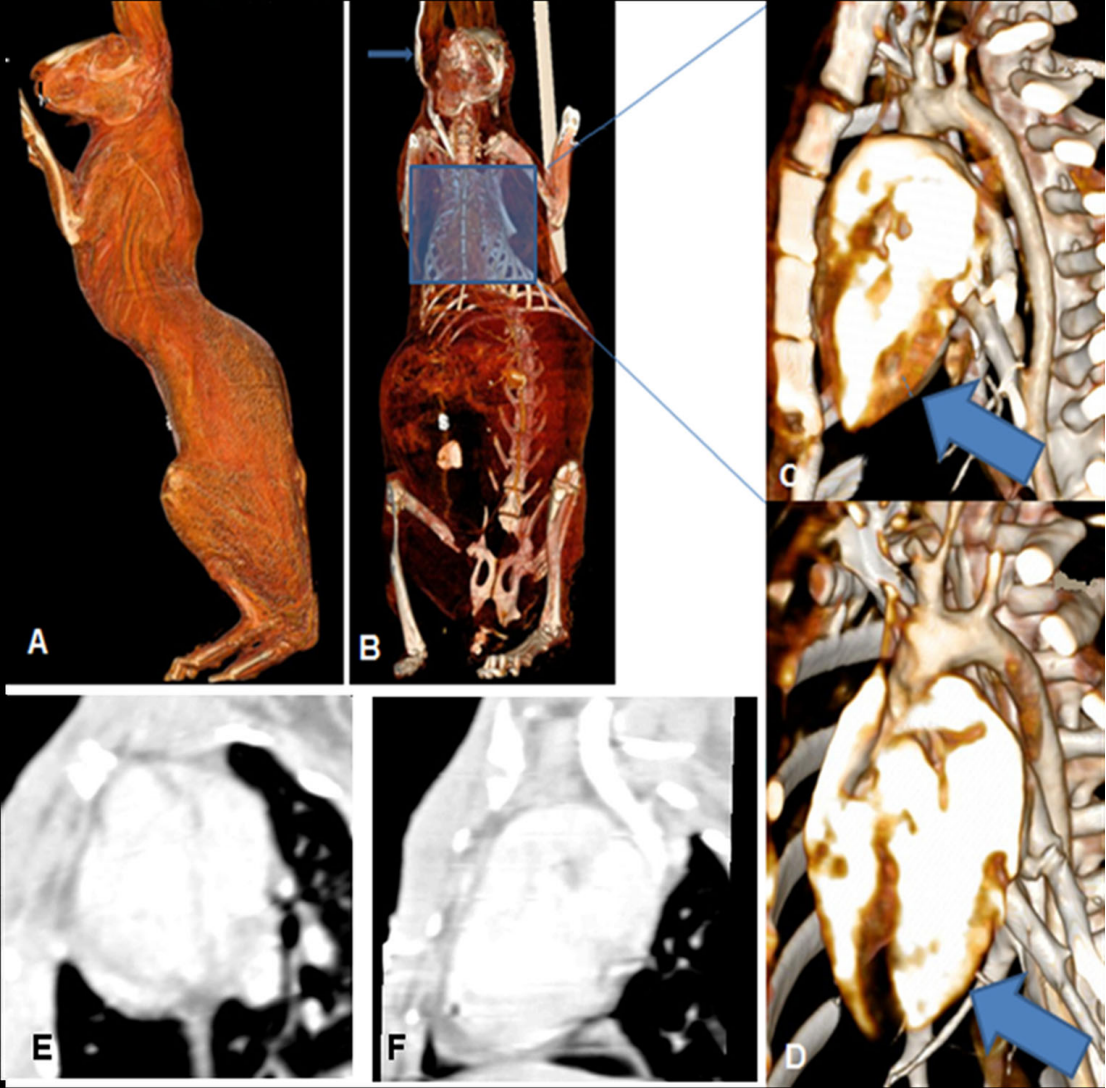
Volume-rendering CT reconstructions. Positioning of the subject (a, b). Normal myocardial wall thickness (*arrow*) before coronary ligature (c). After posterolateral artery ligature, ischemic area shows a marked reduction in ventricular thickness (d).Short axis (e) and long axis (f) scans showing impaired perfusion in the apex and in the lateral wall
